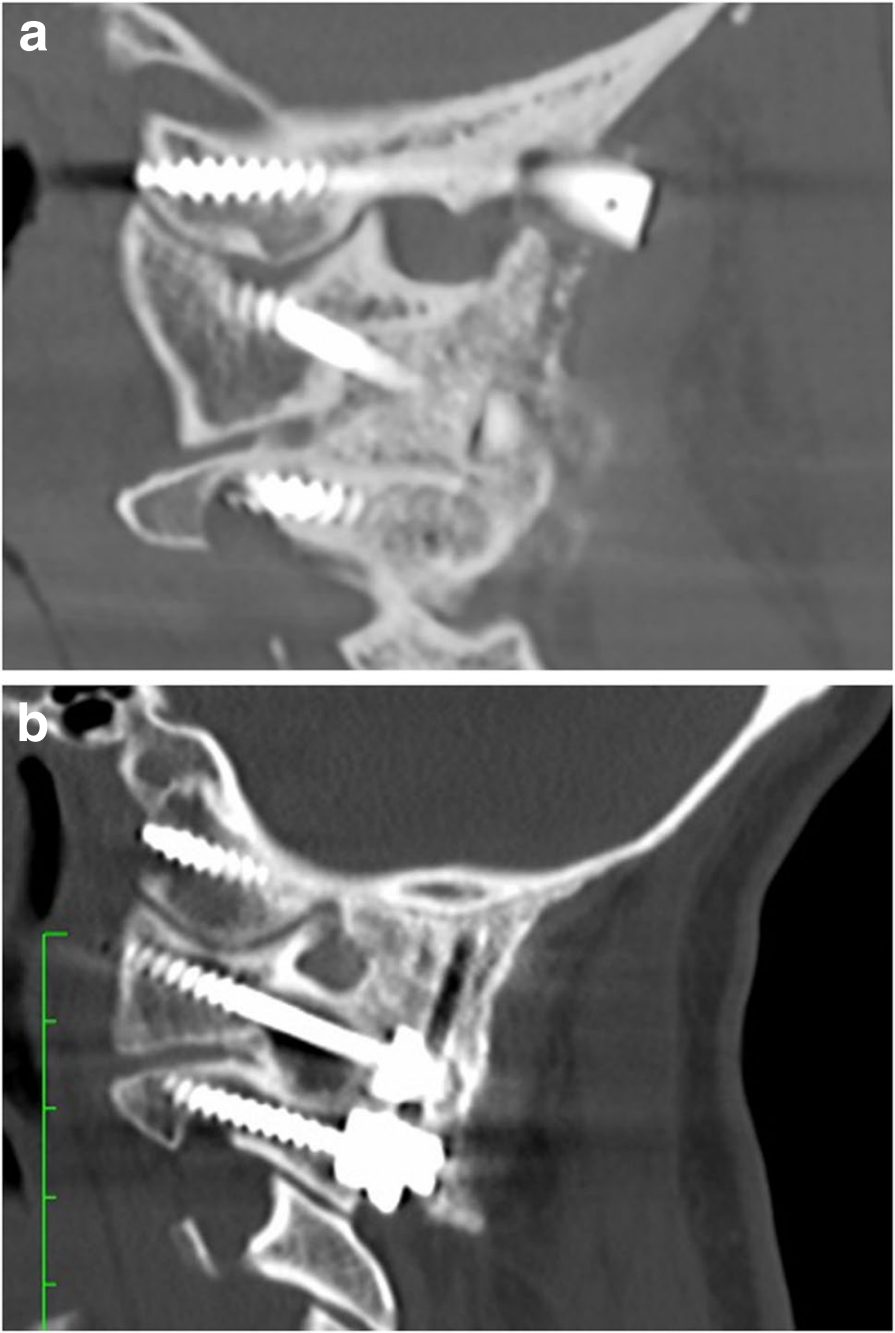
A Sagittal 2D CT showing condylar screw in hypoplastic condyle. B Sagittal 2D CT with C0-2 construct with condylar screws and extremely thin supraocciput
